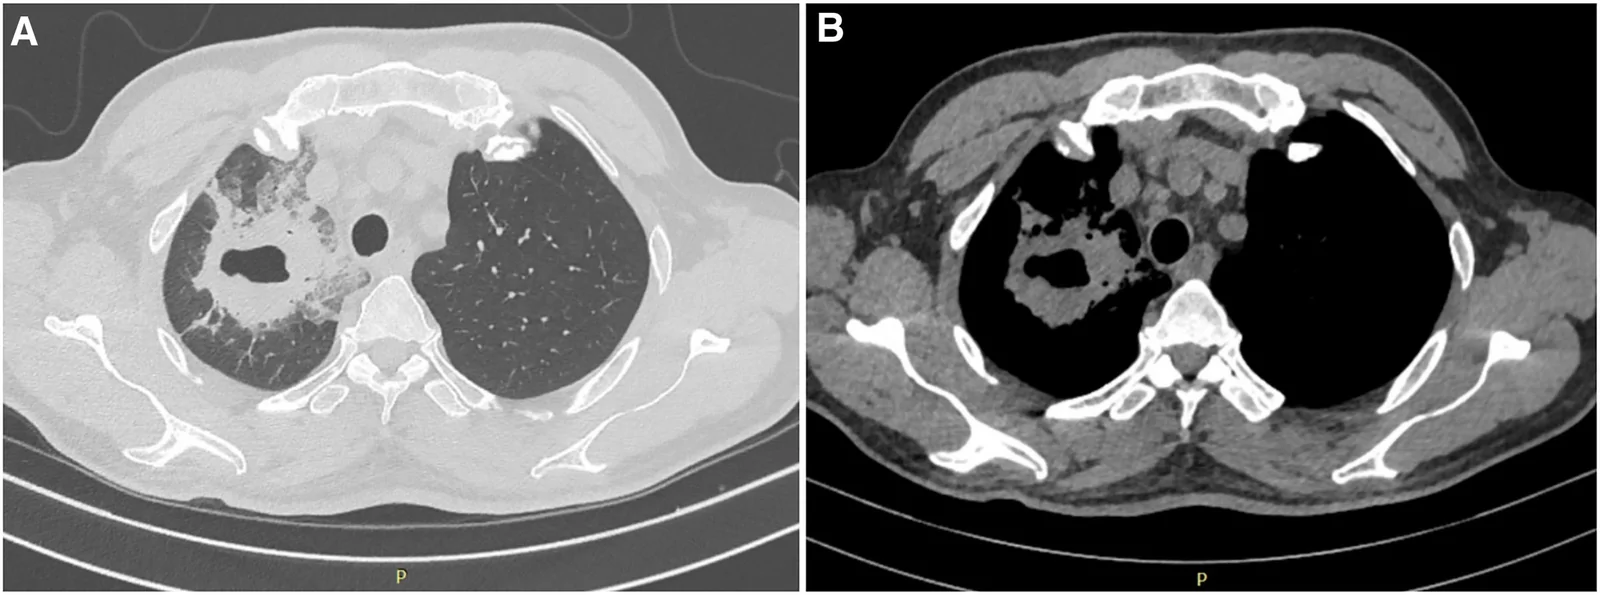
16-slice computed tomographic scan revealed that a cavity lesion in the upper lobe of the right lung, the lesion size was about 9 × 6 cm and the cavity size was about 3.5 × 2.5 cm. (A) lung window; (B) mediation window.
